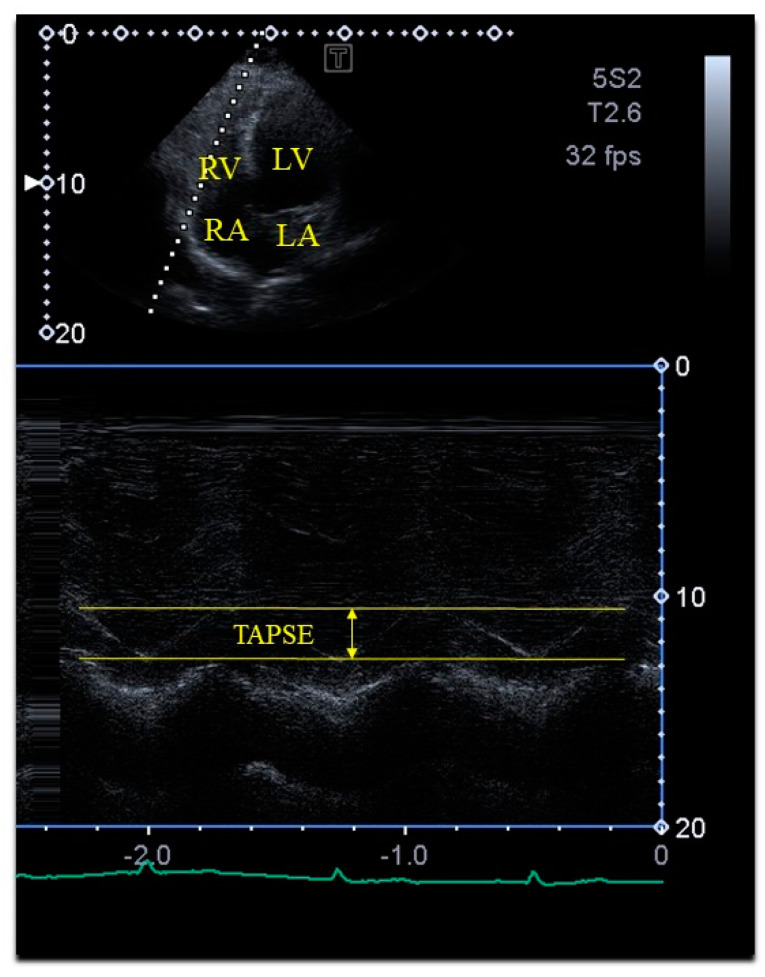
Measurement of tricuspid annular plane systolic excursion (TAPSE) by M-mode echocardiography in apical four-chamber view. Abbreviations: LA = left atrium; LV = left ventricle; RA = right atrium; RV = right ventricle; TAPSE = tricuspid annular plane systolic excursion.
